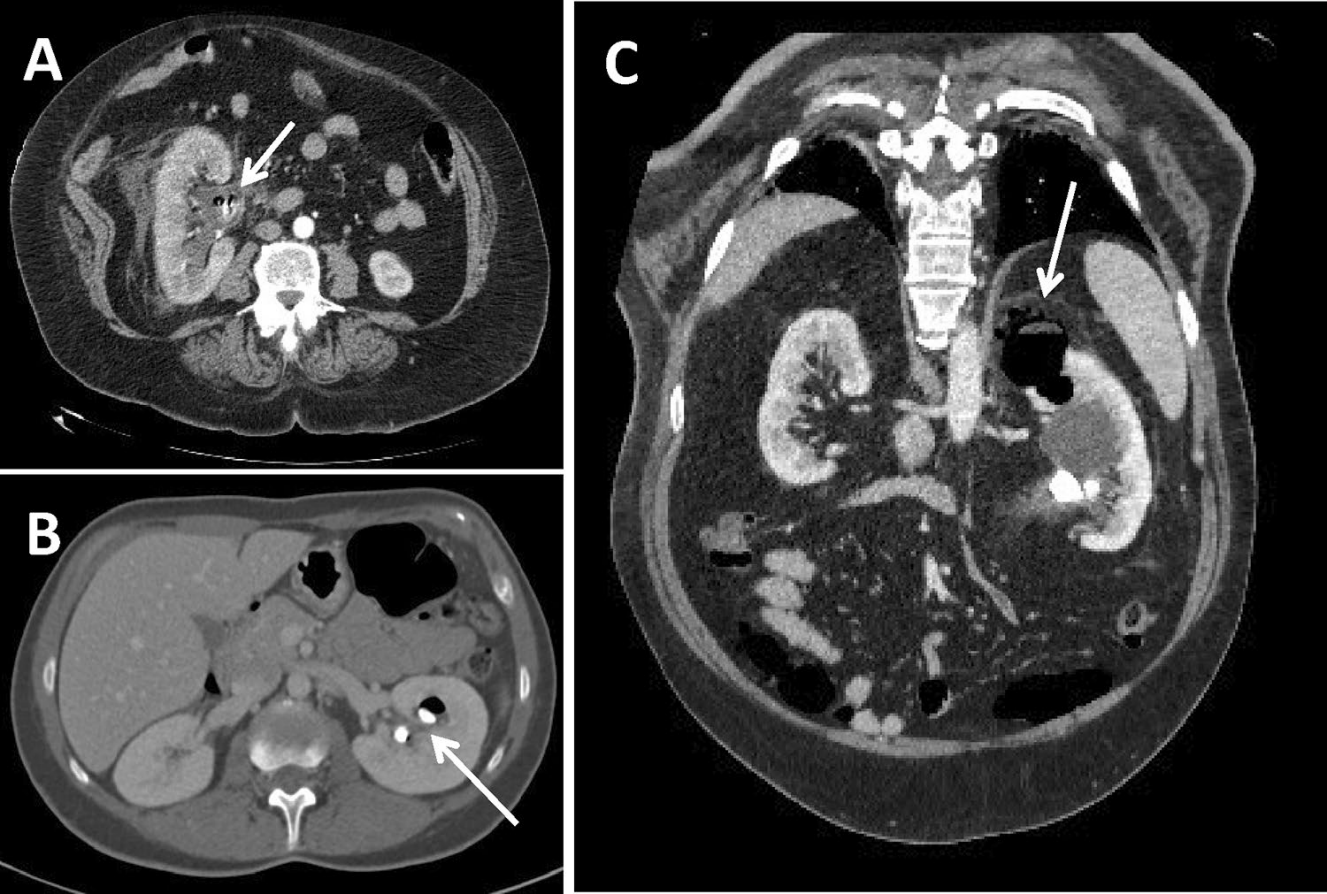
CT staging of emphysematous pyelonephritis. a Axial oblique MPR reconstruction of the parenchymal phase of a 53-year-old diabetic female patient presenting with mild fever shows a class I EPN, characterized by gas only inside collecting system, in association with the presence of a calculus in the renal pelvis (arrow). b Axial scan of the parenchymal phase of a 73-year-old diabetic female patient presenting with right flank discomfort shows two calyceal calculi with initial parenchyma spread of gas (arrow) in a class II EPN. c Coronal oblique MPR reconstruction of a 66-year-old diabetic female patient presenting with abdominal pain shows a class III EPN with a voluminous hypodense fluid-gaseous abscess filled with gas bubbles, dissecting the interstitial space and invading the perinephric space (arrow)
